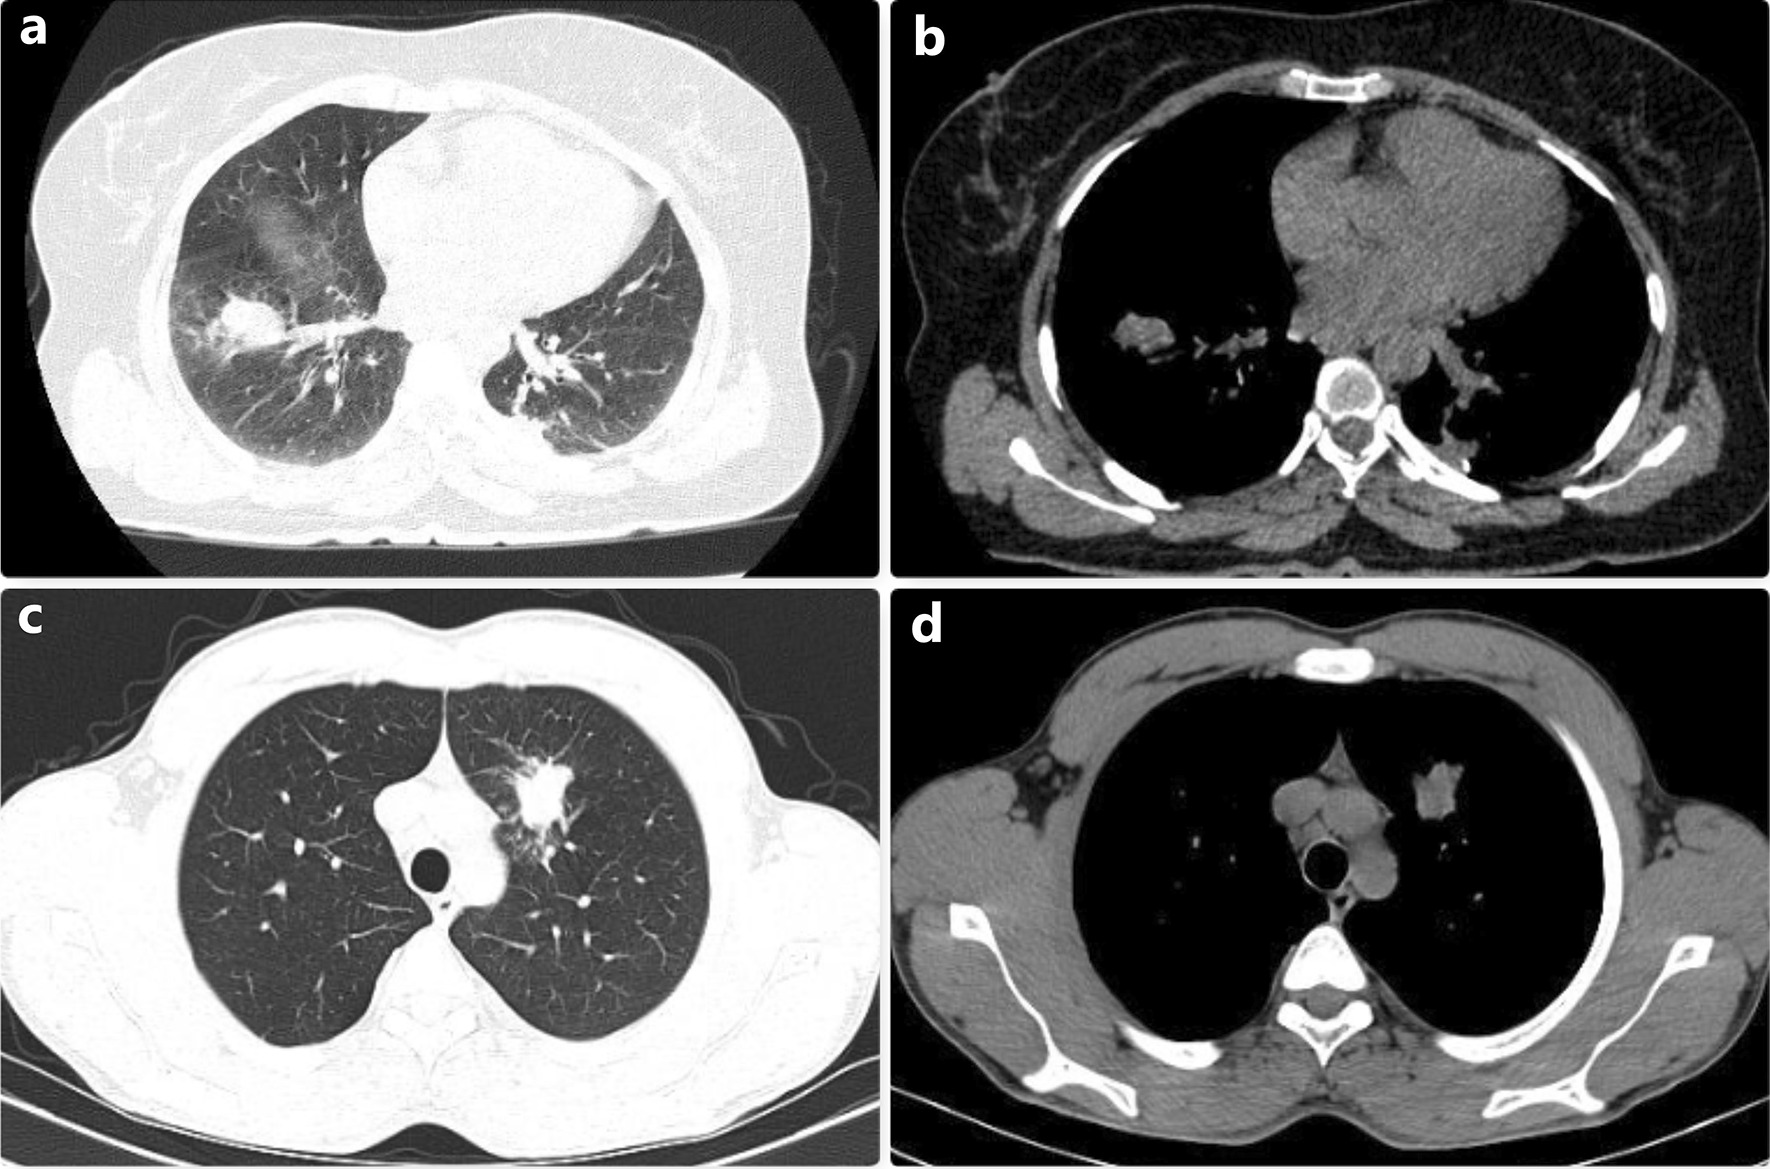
Thoracic CT images of two patients who were operated with a preliminary diagnosis of lung cancer due to heterogeneous opacity in the lung are observed. As a result of the biopsies performed during the operation, it was identified that these images belonged to the perforated hydatid cyst
